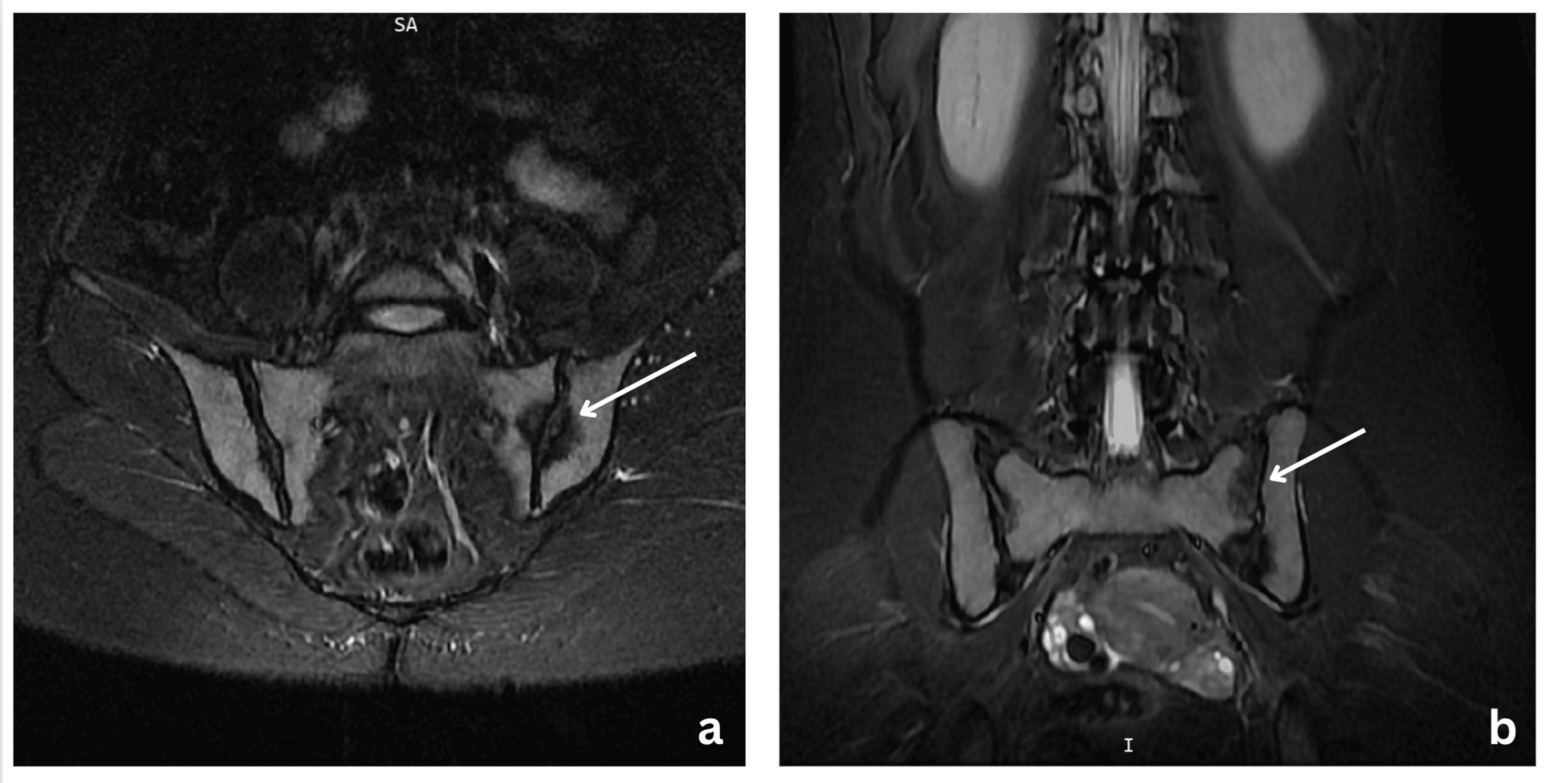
MRI images showing bilateral sacroiliitis. Axial (a) and coronal (b) short tau inversion recovery MRI sequences of the pelvis demonstrate bilateral sacroiliac joint inflammation characterized by hyperintense signal in the joint spaces and surrounding marrow edema. These findings are consistent with active sacroiliitis MRI: magnetic resonance imaging
